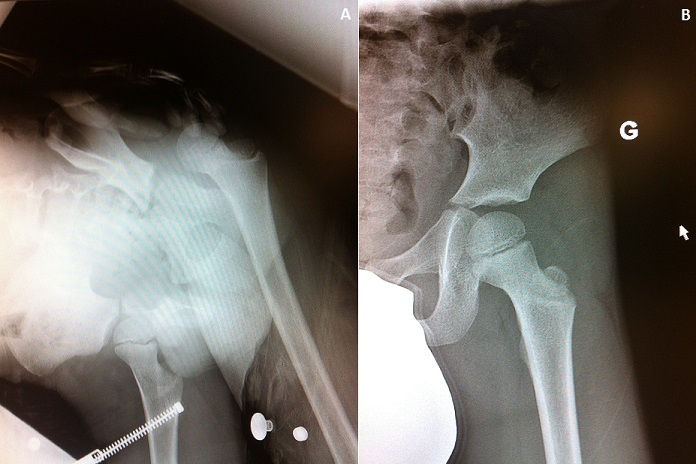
(A) radiographie du bassin montrant la luxation postérieure de la hanche; (B): radiographie du bassin de contrôle montrant la réduction de la luxation
